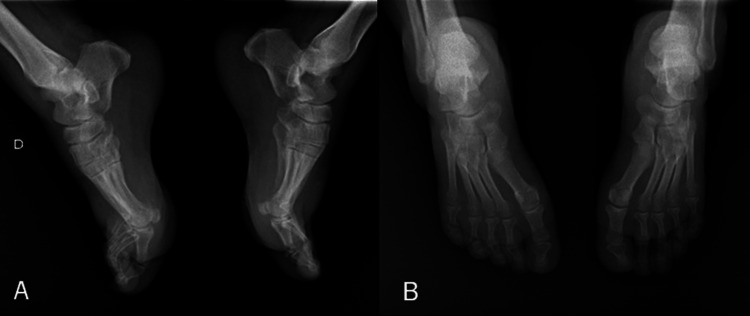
Anteroposterior and lateral radiographs of the left and right without abnormalities (A) Posteroanterior X-ray; (B) Lateral X-ray
